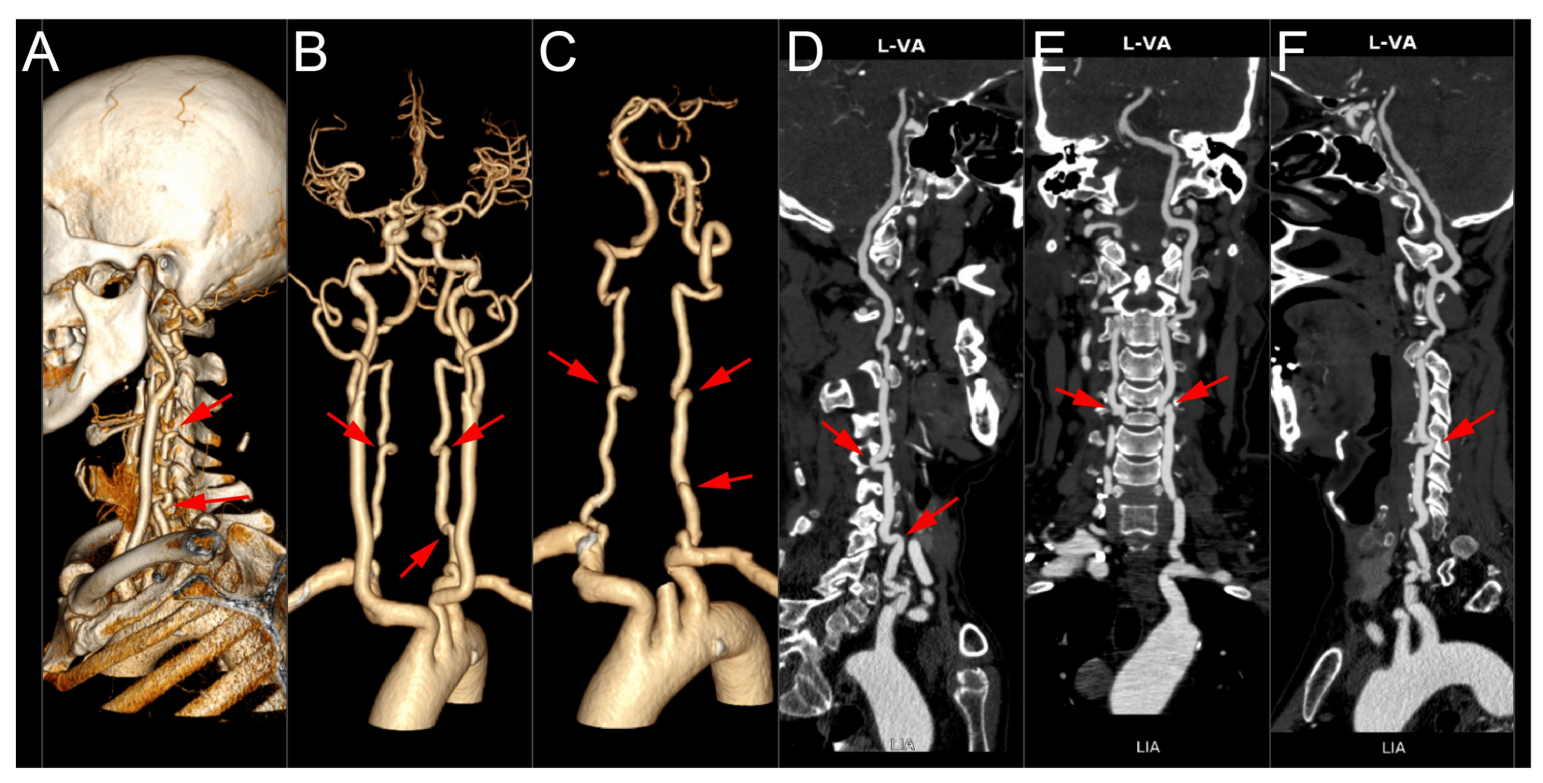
Computed tomography angiography revealing bilateral VALF at the C4-5 level and an additional VALF at the left vertebral artery V1 segment (red arrows) Panels A-F show multiplanar reconstructions demonstrating the tortuous course of the vertebral arteries and their proximity to neural structures. VALF: vertebral artery loop formation
